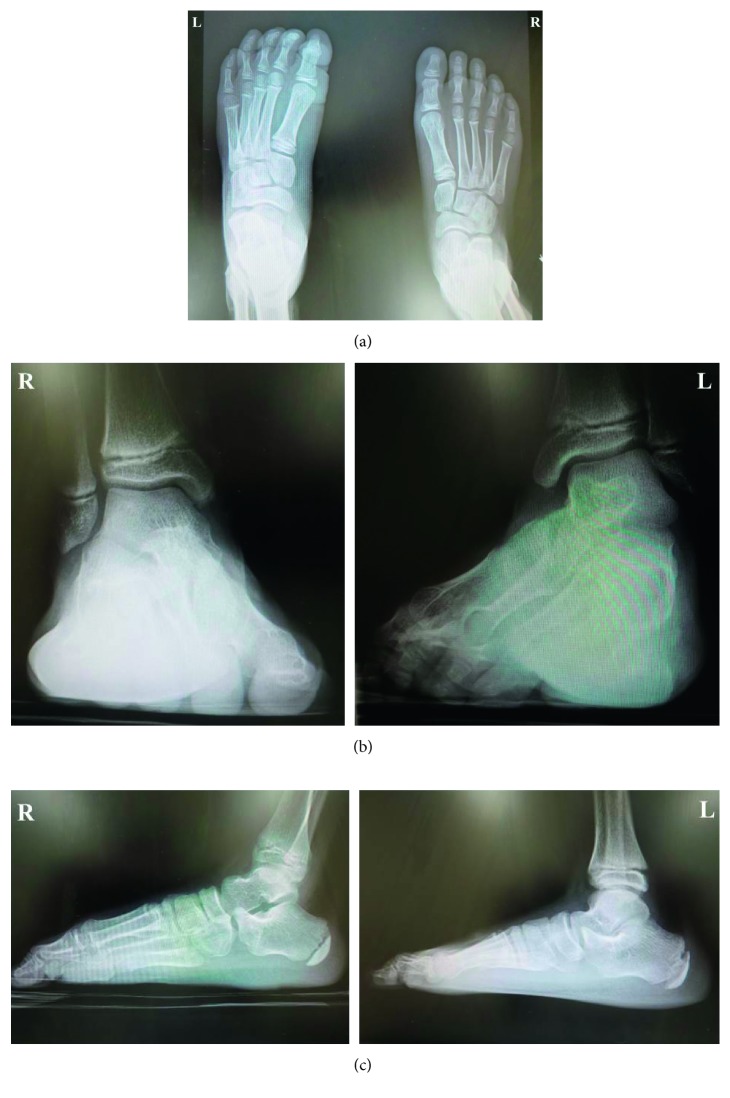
Plain weight-bearing radiographs reveal inversion of the left forefoot on (a) dorsoplantar view and (b) anteroposterior view. (c) Lateral view showing incongruity of the Chopart joint complex.
